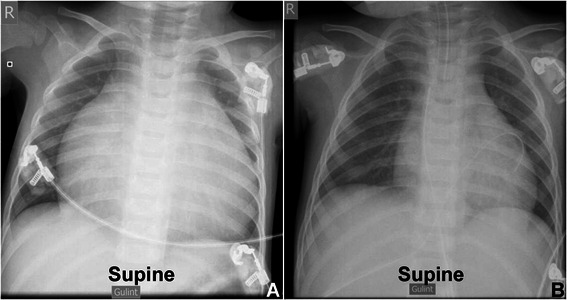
Pre-operative and Post-operative chest X-rays. a – Anteroposterior chest X-ray performed in supine position. Extreme cardiomegaly is visible. b – Anteroposterior chest X-ray performed in supine position, performed 12 h after surgery. Slight cardiomegaly is visible
